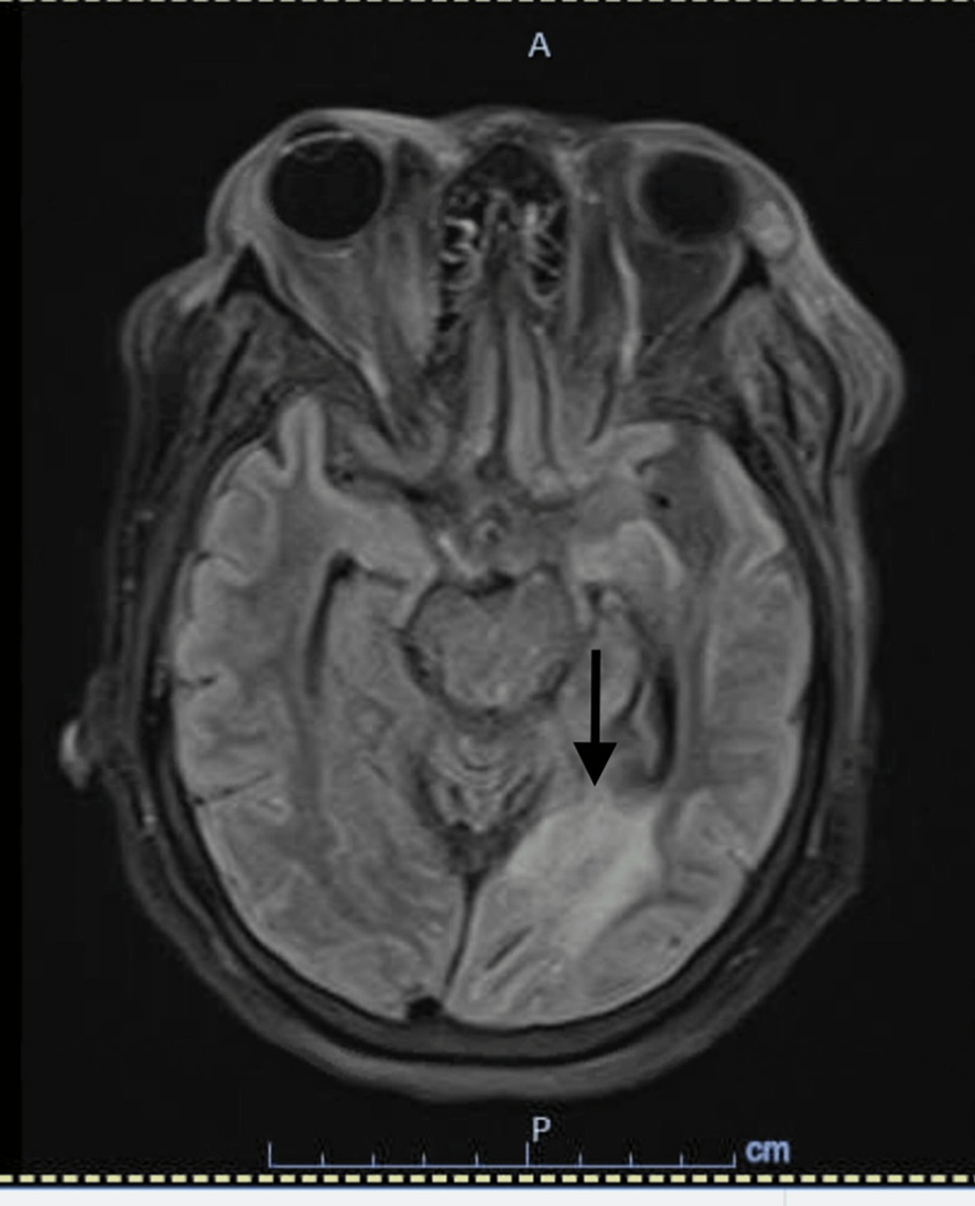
Relative improvement of fluid attenuation inversion recovery (FLAIR) changes on MRI brain MRI brain showing improvement in left posterior parietal and occipital lobes hyperintensity in FLAIR sequence (arrow).
